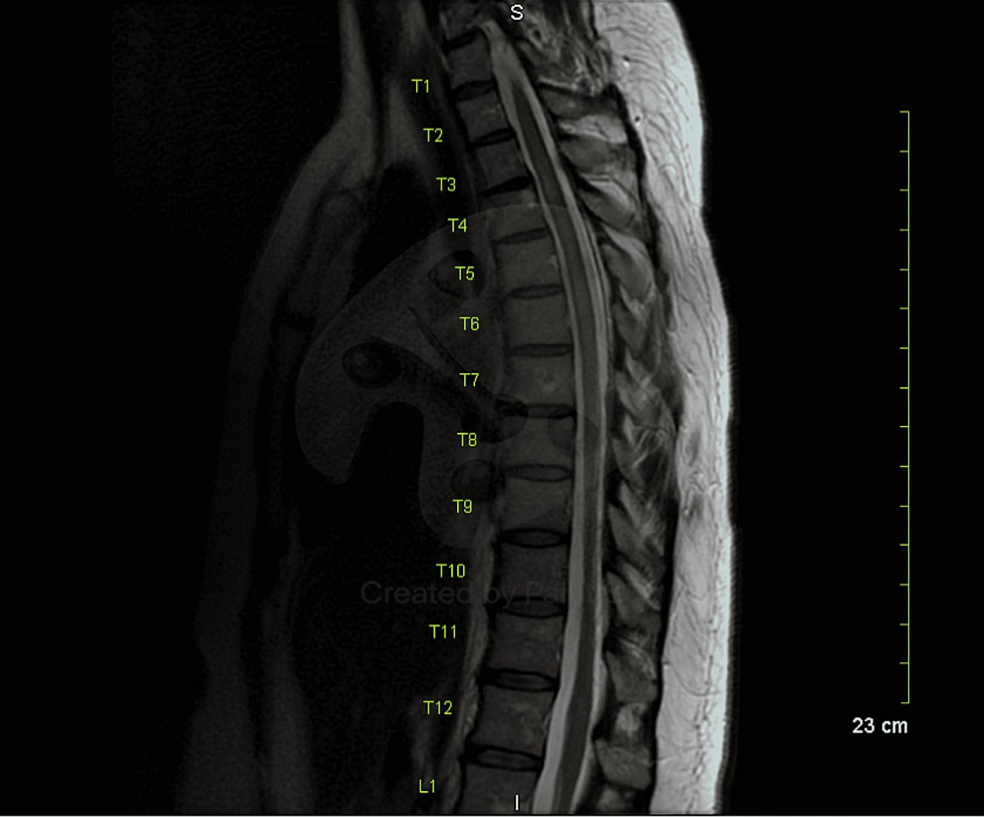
T2 MRI thoracic spine with and without contrast shows resolution of demyelination lesions and no new lesions were found.
